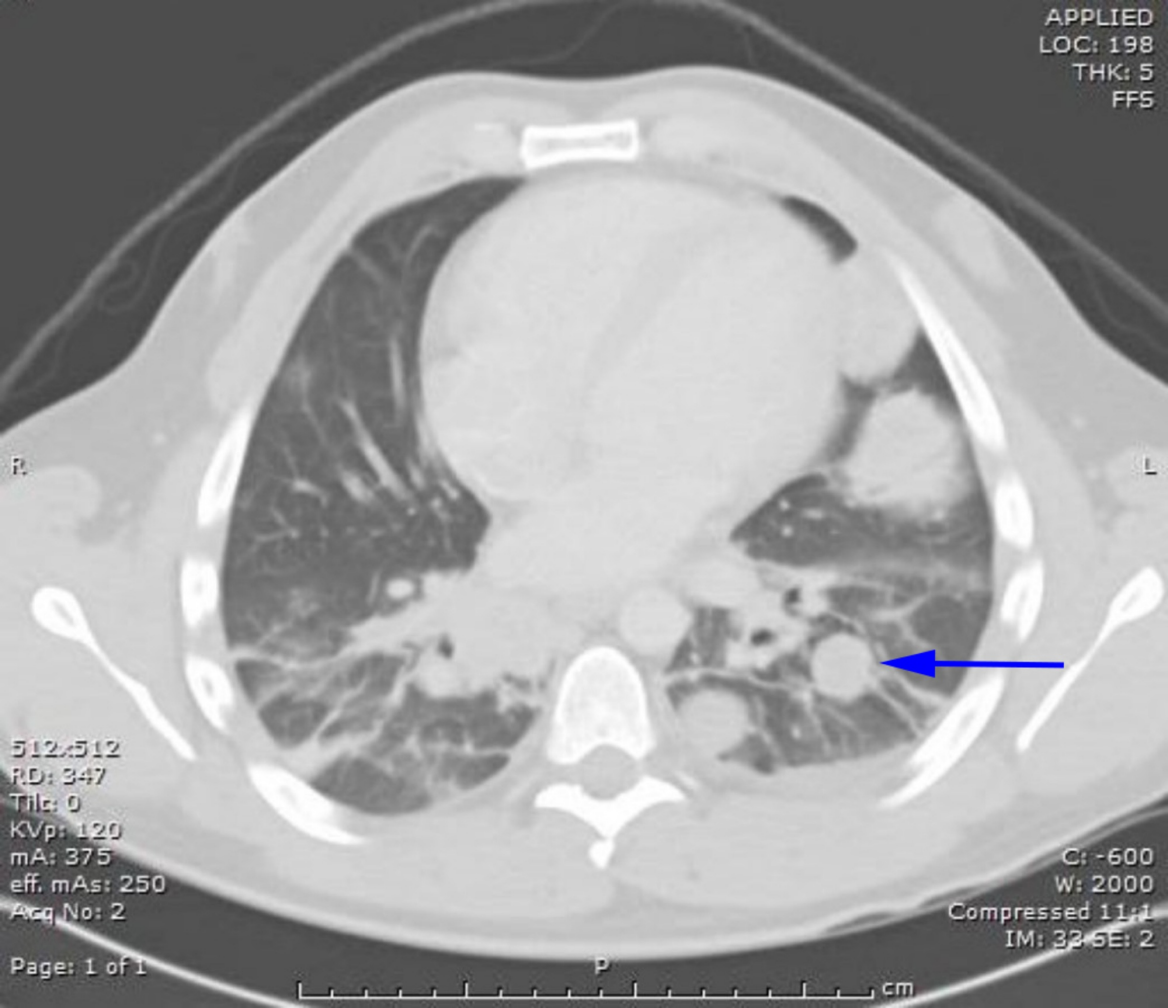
Computed tomography (CT) of the chest with multiple lung metastases CT of the chest showing multiple metastatic lesions in lungs.
